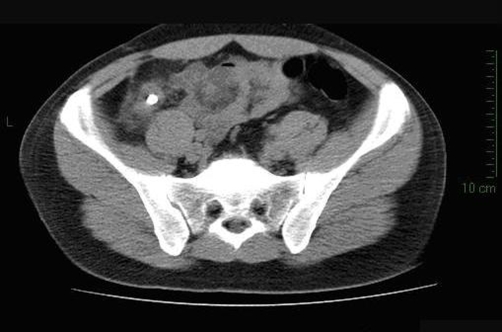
Computed Tomography scan showing a hyperdense formation in RLQ surrounded by an
